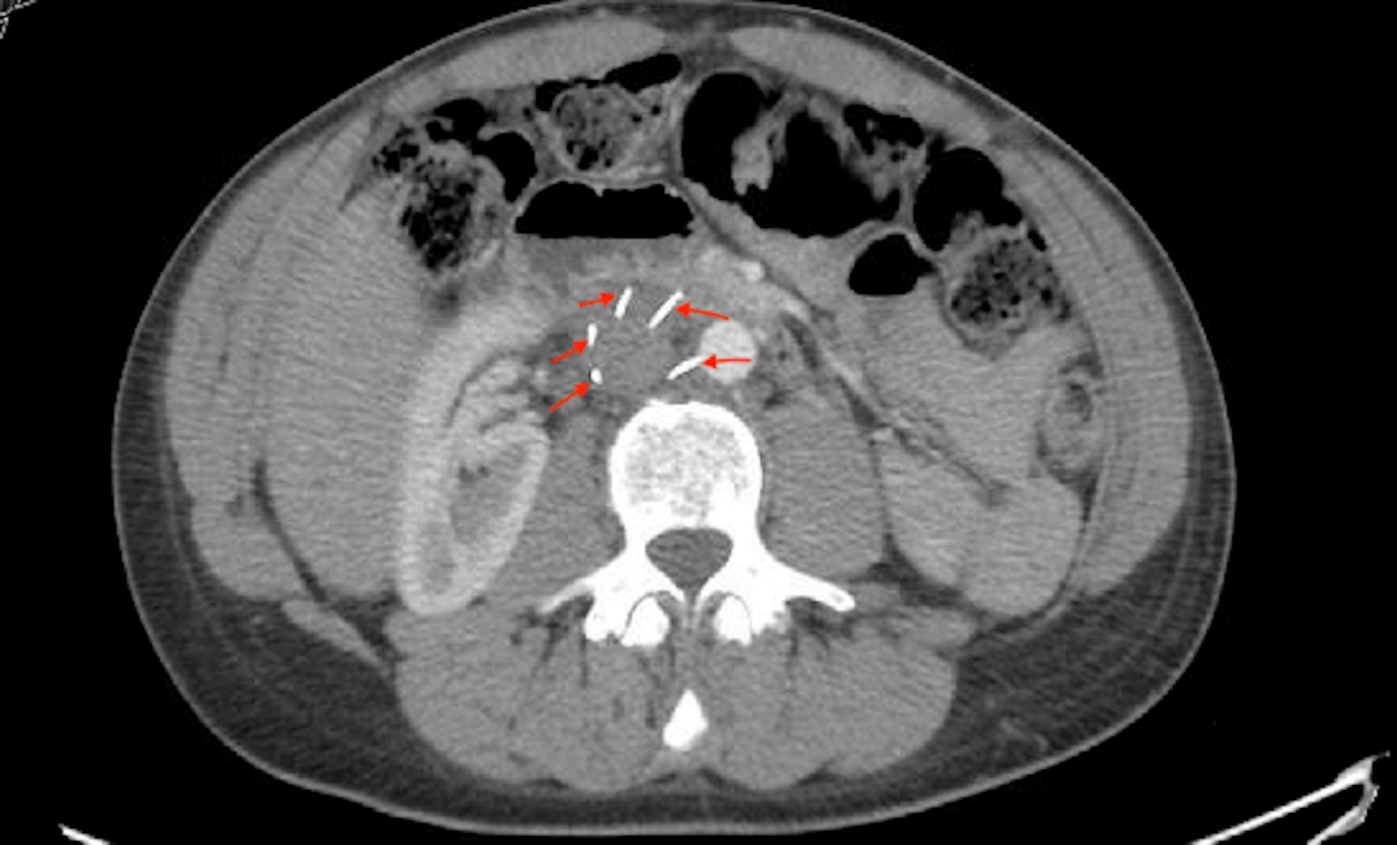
CT of the abdomen showing only five out of the six struts of the inferior vena cava filter with one strut missing.
